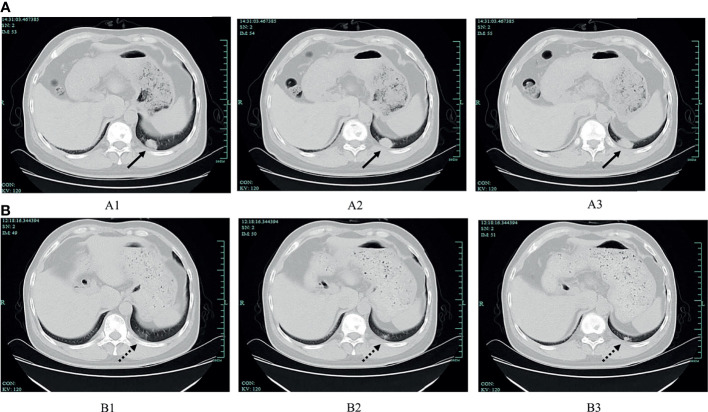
The comparison of chest CT before and after sintilimab treatment. (A) Chest CT scan before sintilimab treatment (July 2020). Images of A1, A2, and A3 consecutively showing the tumour at the posterior segment of the left lower lobe, and the tumour size was 2.1×1.4 cm, 2.4×1.5 cm, 2.7×1.5 cm, respectively (solid lines indicate the tumour). (B) Chest CT scan after sintilimab treatment (September 2020). Images of B1, B2 and B3 showing the tumour at the same location when compared with A1, A2, and A3, separately, and the tumour size was 0.4×0.4 cm, 0.5×0.4 cm, 1.0×0.7 cm, respectively (dotted lines indicate the tumour).
